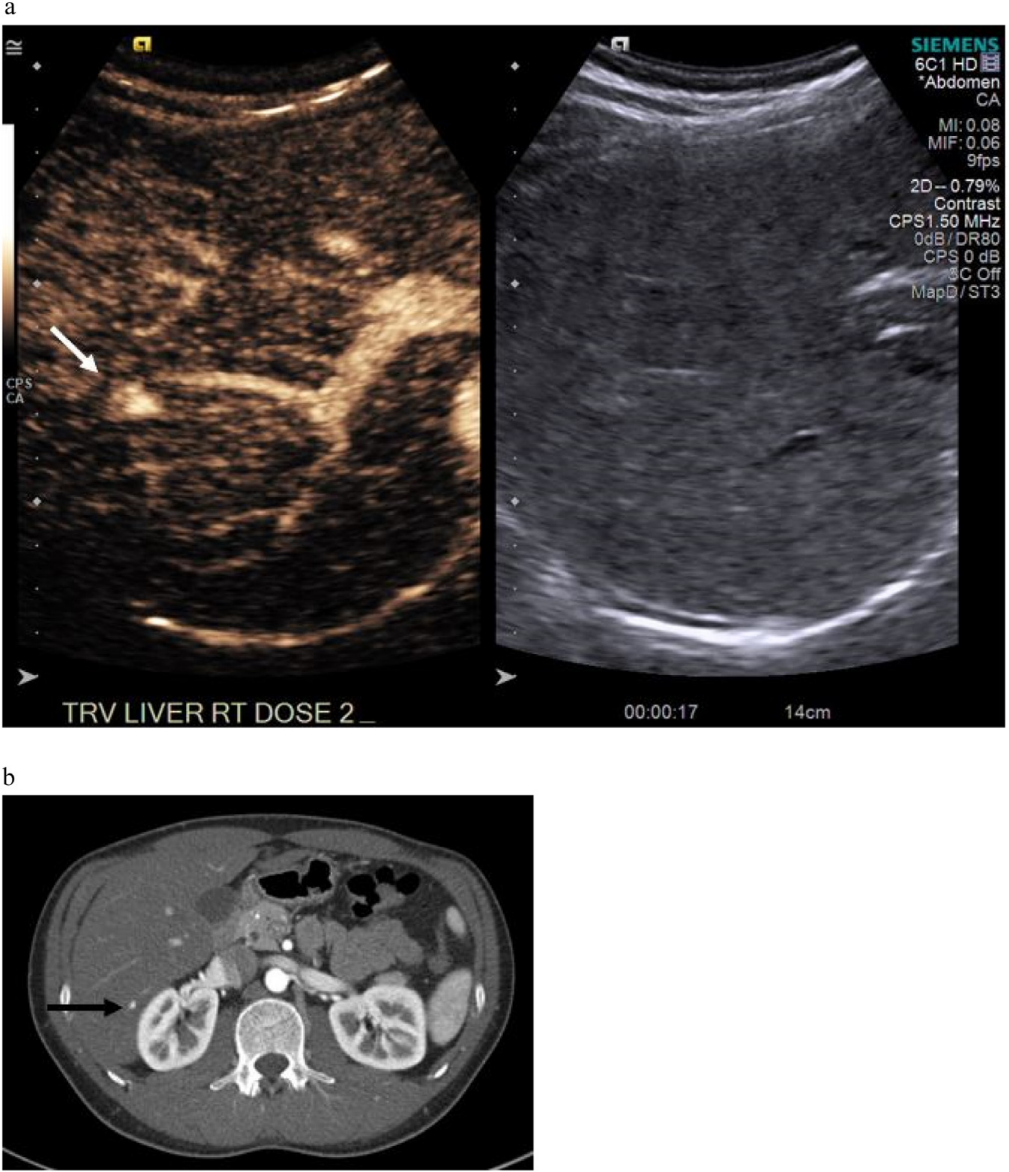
LIRADS 4 lesion that was downgraded on follow-up CT. A 1.1 cm lesion is seen on during arterial phase sweeps (a) denoted by white arrow. No washout on CEUS (not shown), and downgraded to LIRADS 3 on CT. (b) Arterial phase CT imaging shows the correlate lesion (black arrow), which was stable on 1.5 years of subsequent follow-up.
